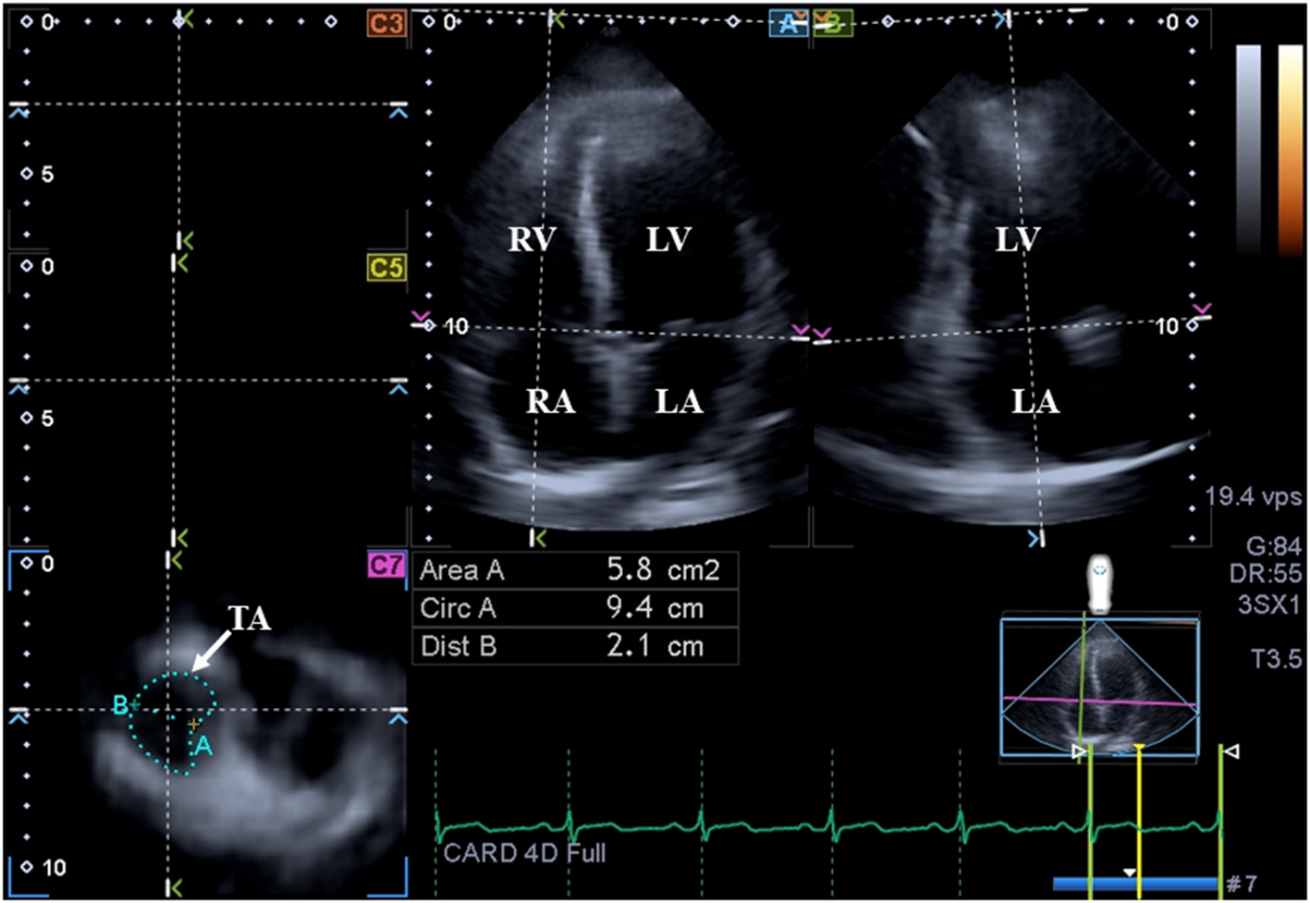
Assessment of the tricuspid annulus is presented extracted from a three-dimensional full-volume dataset: apical four-chamber view (A); apical two-chamber view (B); and a cross-sectional view at the level of the tricuspid annulus optimized in apical four- and two-chamber views (C7). The white arrow represents the tricuspid annular plane. LA, left atrium; LV, left ventricle; TA, tricuspid annulus; RA, right atrium; RV, right ventricle; Area, TA area; Circ, TA perimeter; Dist, TA diameter.
